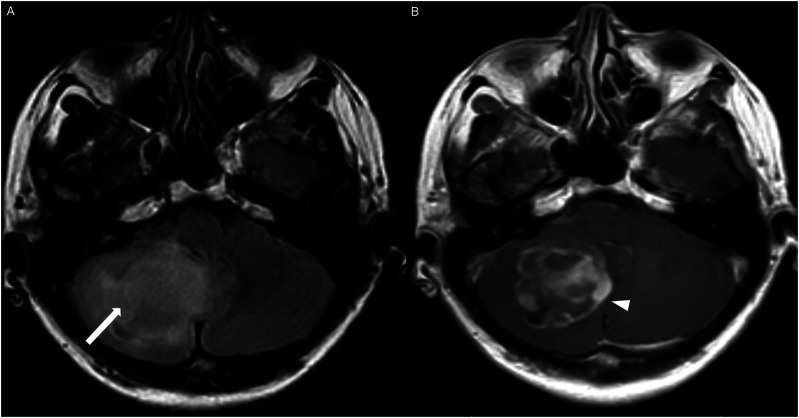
Axial FLAIR (A) and axial post-contrast T1 weighted images (B), show a FLAIR hyperintense mass involving the right cerebellum (A, arrow), demonstrating heterogeneous enhancement (B, arrowhead). There is surrounding edema, mass effect and partial effacement of the fourth ventricle.
